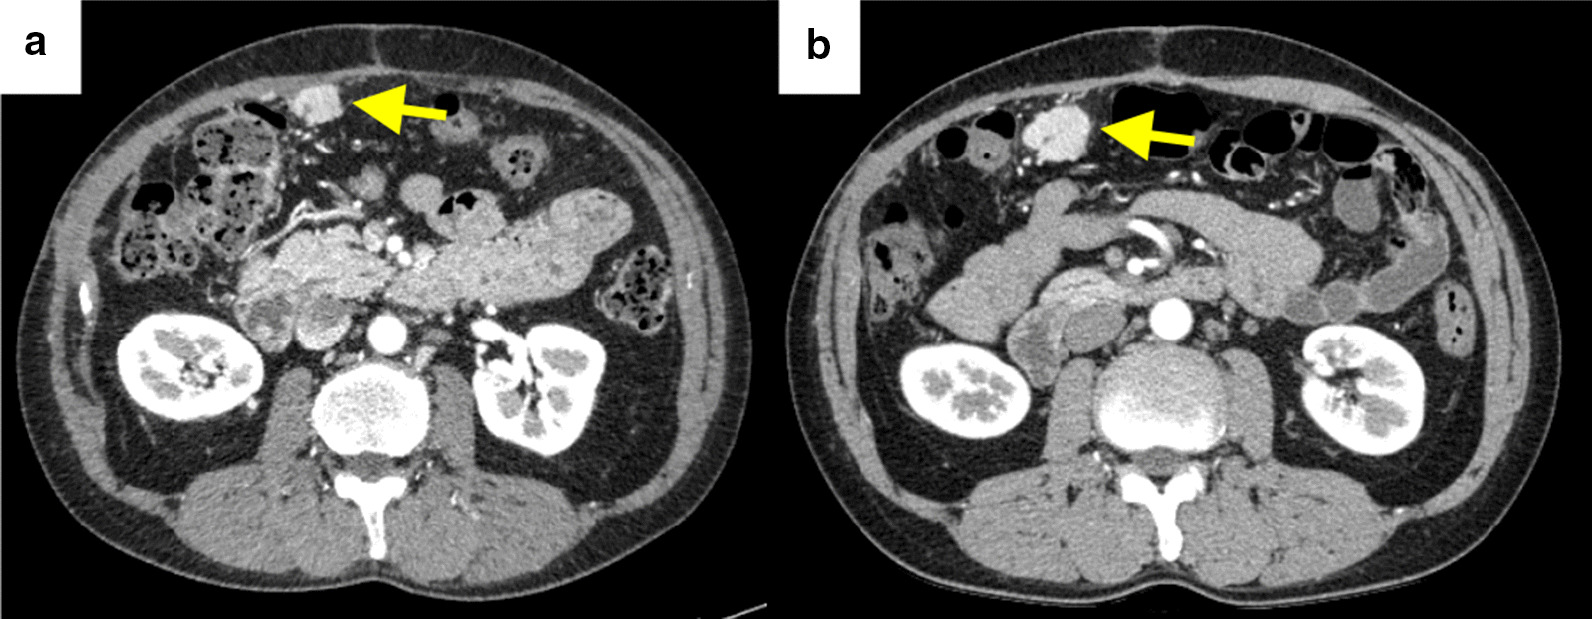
Contrast-enhanced abdominal computed tomography images a Preoperative image showing multiple nodules suspected of denoting peritoneal dissemination. Yellow arrow, biggest nodule, which had a doubling time of 22 days. b Image obtained 3 weeks after that shown in (a)
